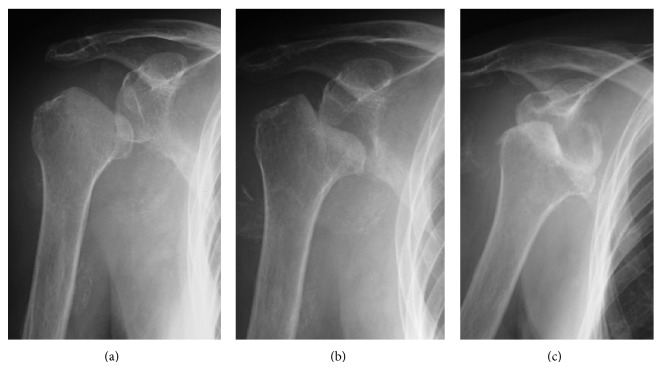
Case  1. Serial preoperative radiographs. (a) At the first visit, the humeral head shows a minor collapse. (b) Three weeks later, the collapse of the humeral head has progressed. (c) Four months later, the destruction of the shoulder is further progressed, including the glenoid.
